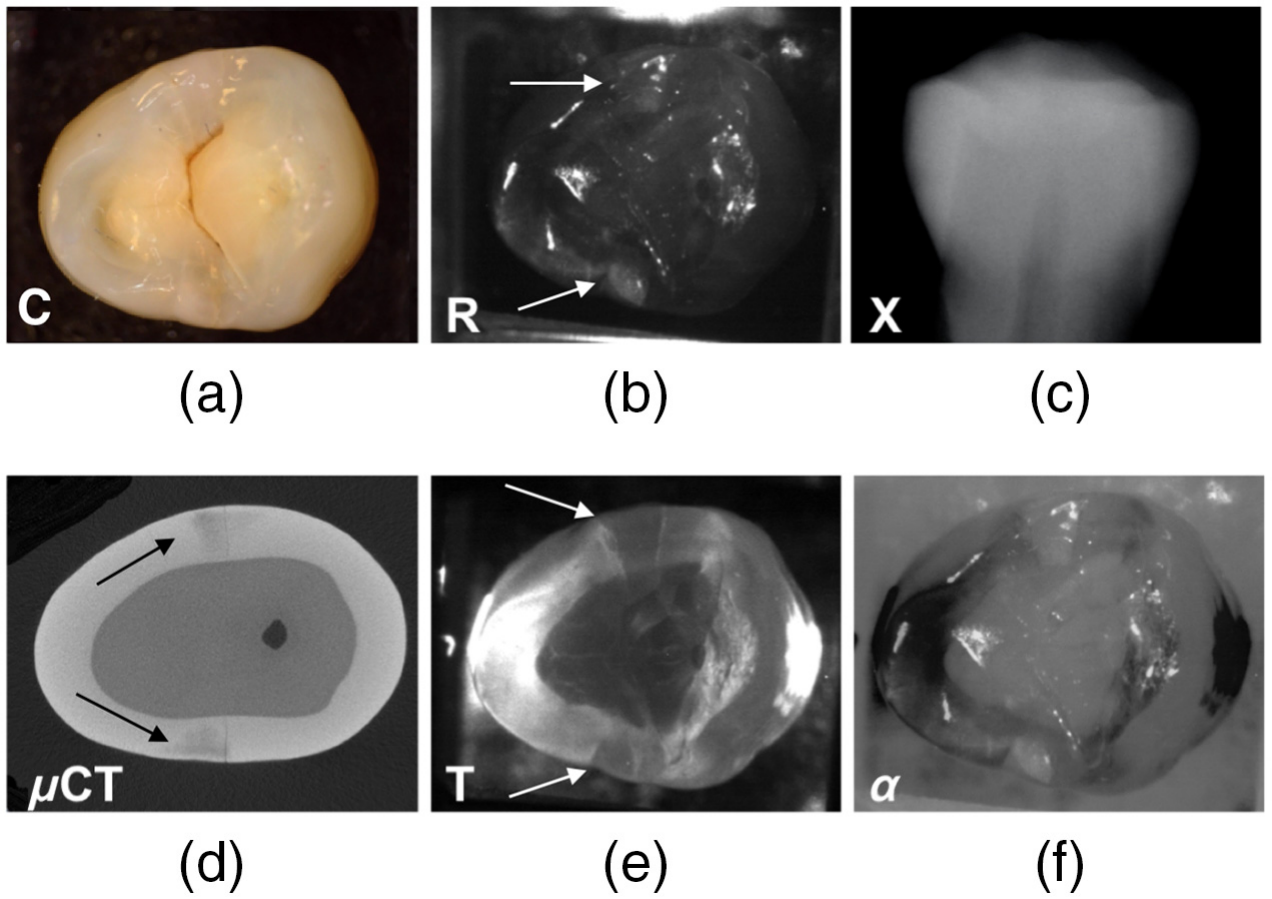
(a) Color; (b) SWIR reflectance at 1600 nm; (c) dental radiographic; (d) μ−CT; (e) SWIR transillumination at 1300 nm; and (f) combined R + T fusion (α=0.4) images of a tooth with two approximal lesions. Arrows point to lesions.
